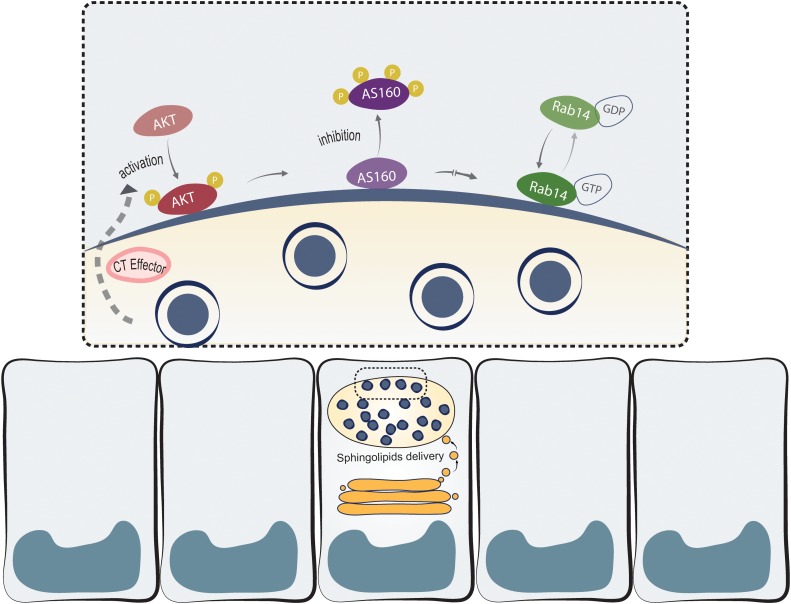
Chlamydia trachomatis* hijacks Akt/AS160/Rab14 cascade to acquire Golgi-derived sphingolipids. *Ct* phosphorylates and recruits pAkt to the inclusion membrane by one or more cognate effectors (CT effector). Upon Akt activation, AS160 is inactivated by phosphorylation and it is detached from the inclusion membrane. Hindered AS160 GAP-activity enables Rab14 to remain in its GTP-bound membrane-associated active form. This, in turn, favors the arrival to chlamydial inclusions of sphingolipids synthesized at the Golgi apparatus through a Rab14-controlled vesicular transport.
